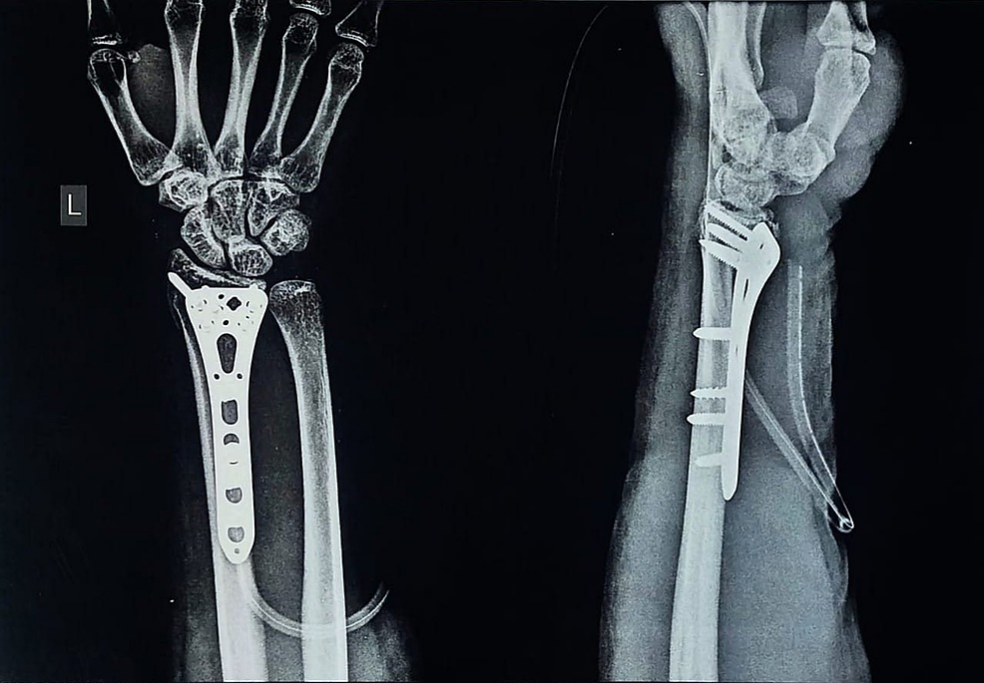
Postoperative X-ray of intra-articular distal end radius fracture treated with locking compression plate.
